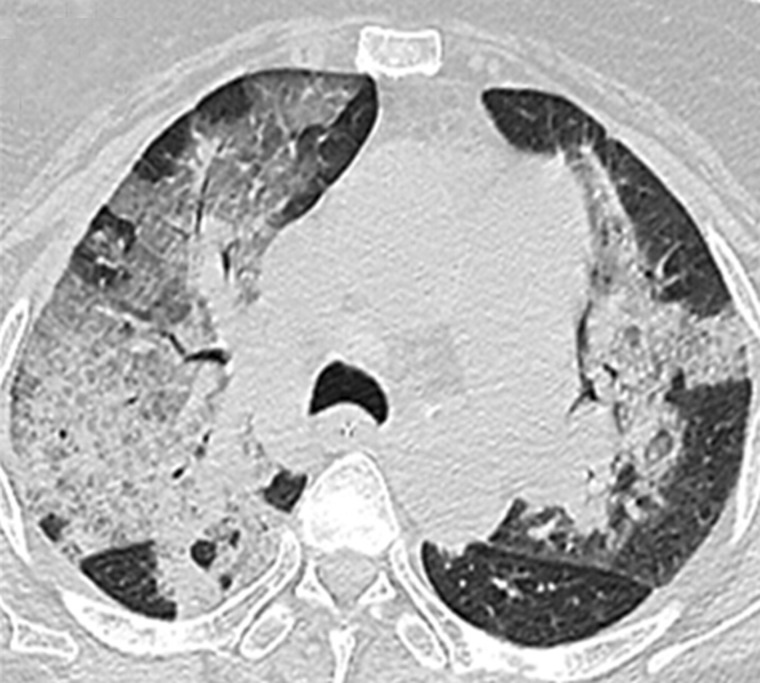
Pulmonary hemorrhage. Axial high resolution CT scans showing consolidation superimposed with ground glass opacities suggestive of pulmonary hemorrhage.
